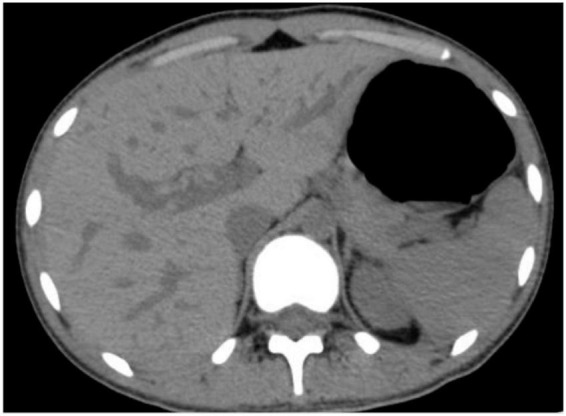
Preoperative abdominal computed tomography showing abnormal density shadows in the right hepatic lobe with possible hemorrhage.
